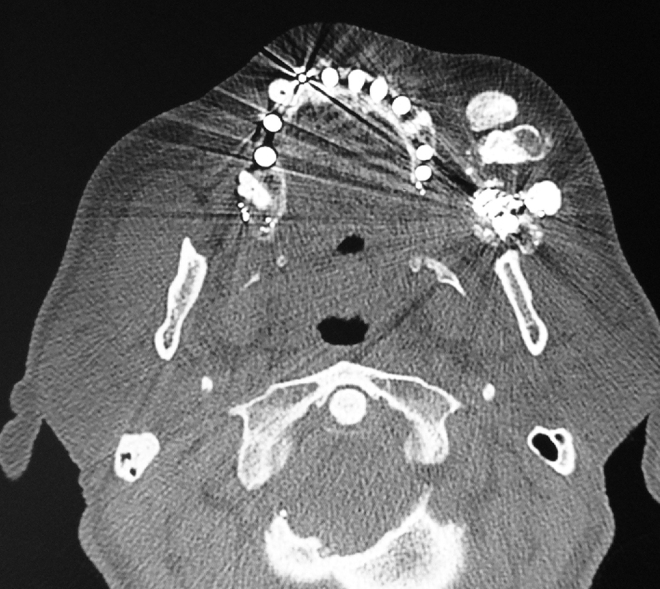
CT scan, bone window. View of three bone lesions and metallic fragments.
